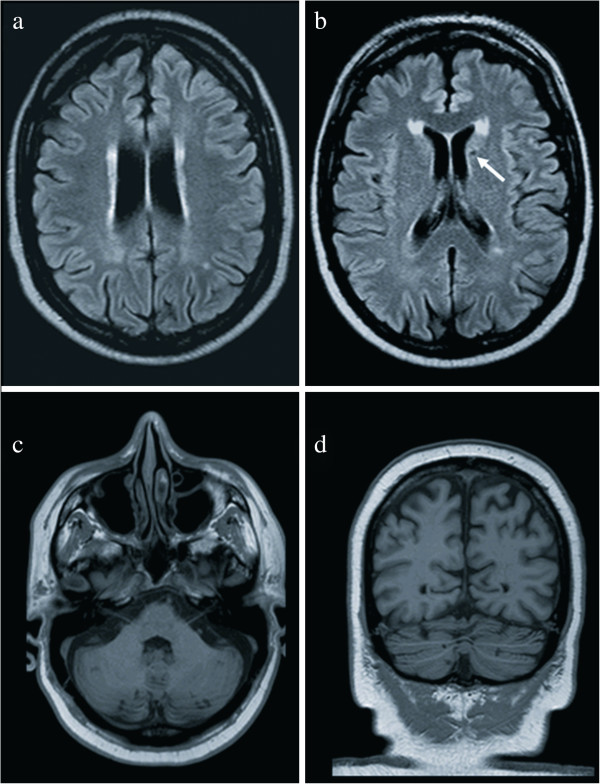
Brain MRI of a 43-year-old male patient. Axial FLAIR (a, b); axial (c) and coronal (d) T1 weighted images. a,b) hyperintense rim bordering the margin of both lateral ventricles and a small lacune in the left head of the caudate (arrow); c, d) multiple small hypointense lesions in the cerebellar cortex.
